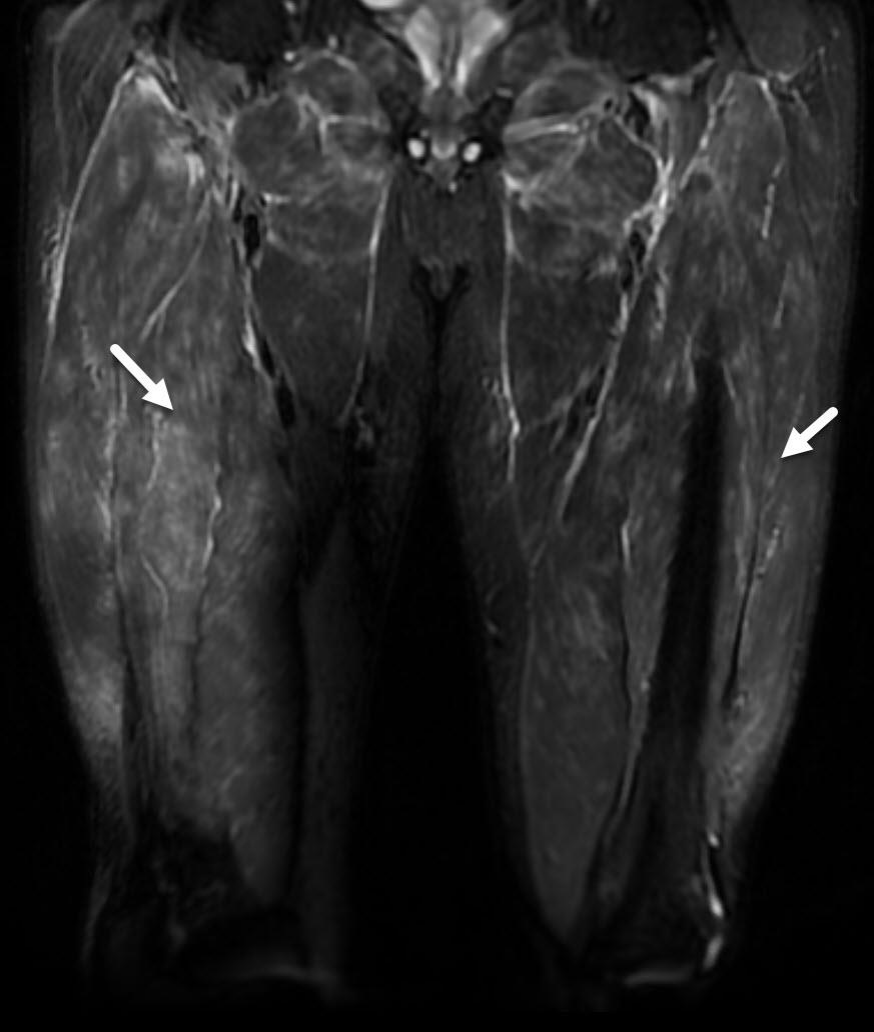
Magnetic Resonance Imaging Scan of the Patient’s Thigh Muscles. Coronal view: short tau inversion sequence (STIR) sequence, showing significant diffuse bilateral muscle edema (arrows).
